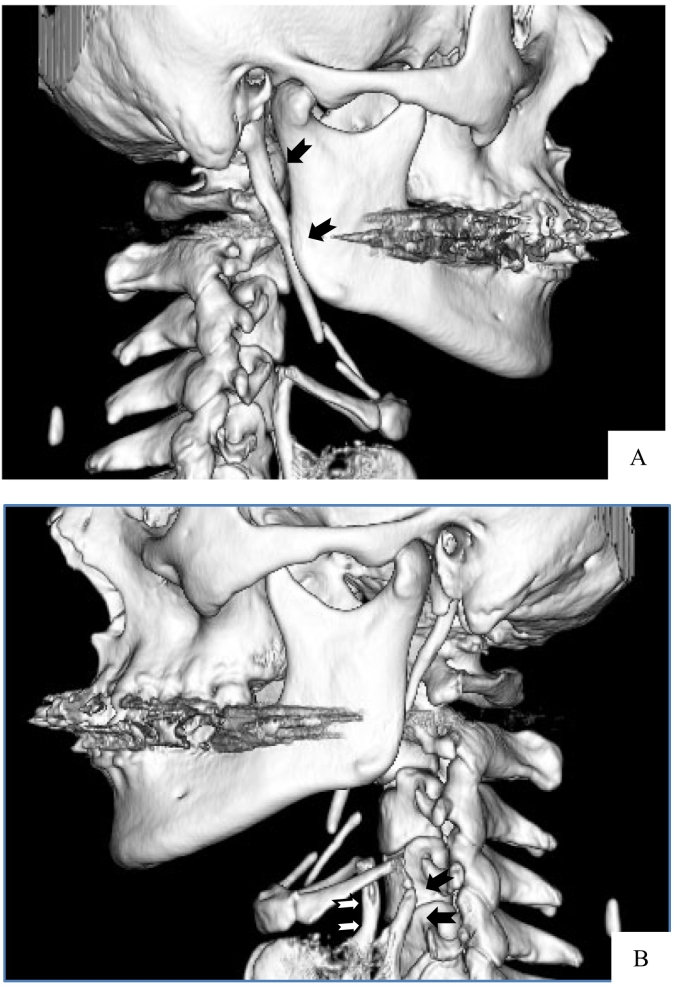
CT image. Prolongation of the styloid process close to the hyoid. Prolongation of the superior cornu of the thyroid cartilage was observed (arrows).
